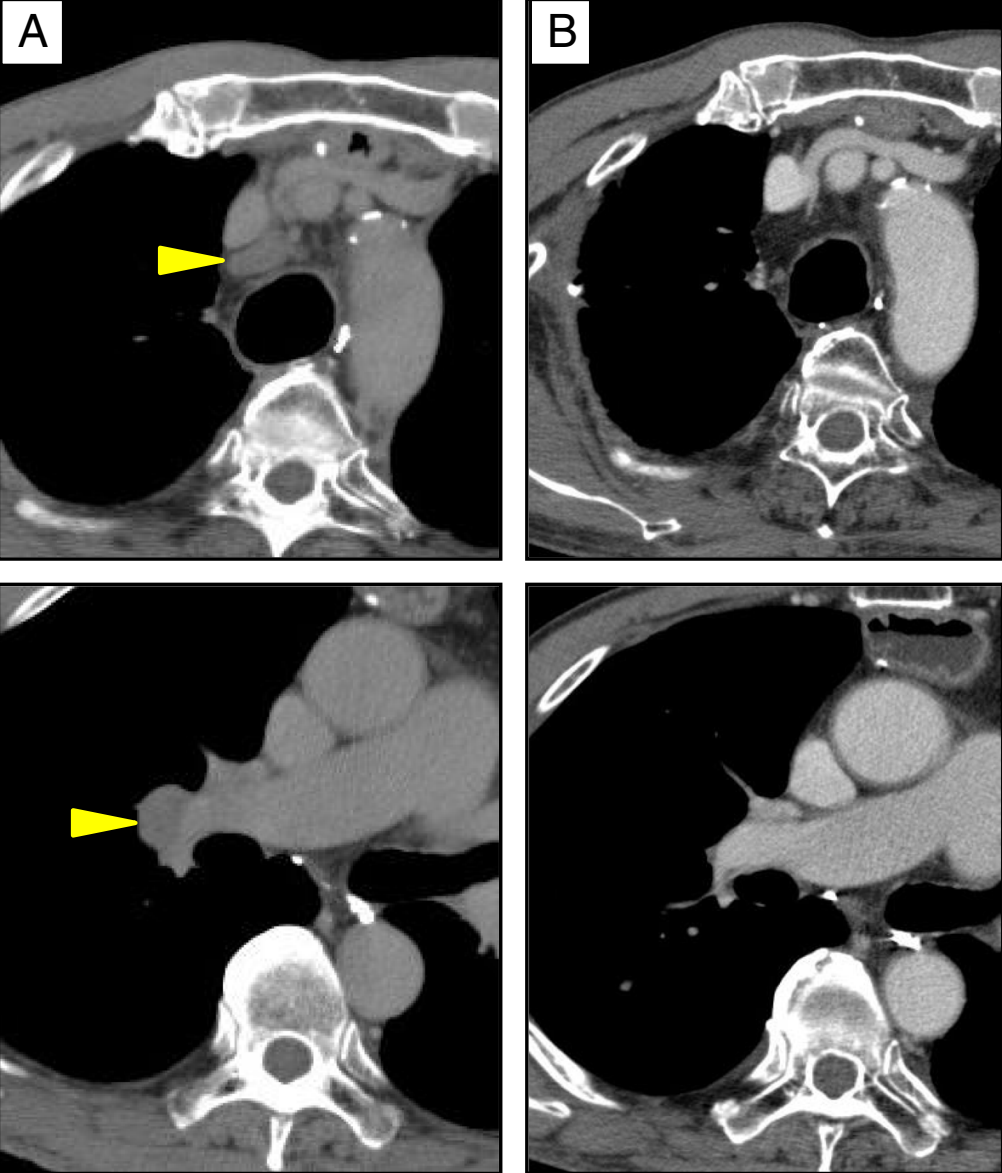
CT images showing LN metastases at mediastinum and hilum. a Before chemotherapy (arrowheads). b After treatment (the LNs have disappeared)
